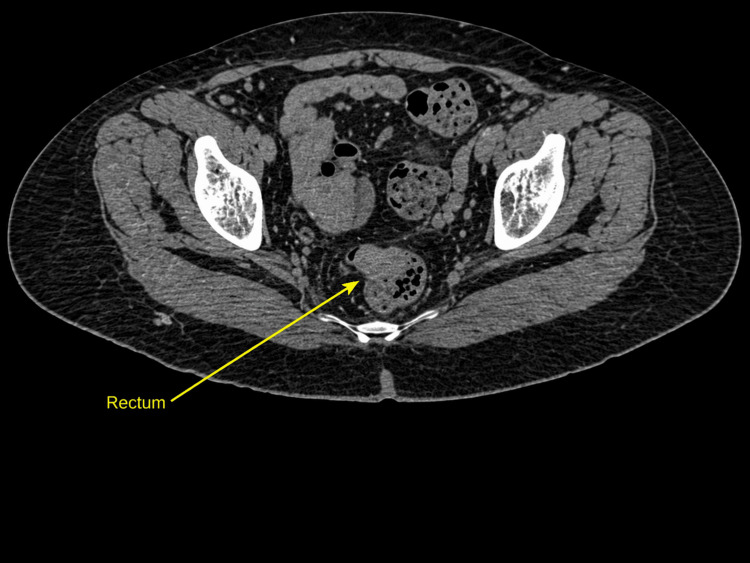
Contrast-enhanced axial pelvic computed tomography demonstrating the rectum (arrow) with no radiological evidence of mesorectal lymphadenopathy, locoregional invasion, or distant metastatic disease, consistent with localized disease at presentation.
